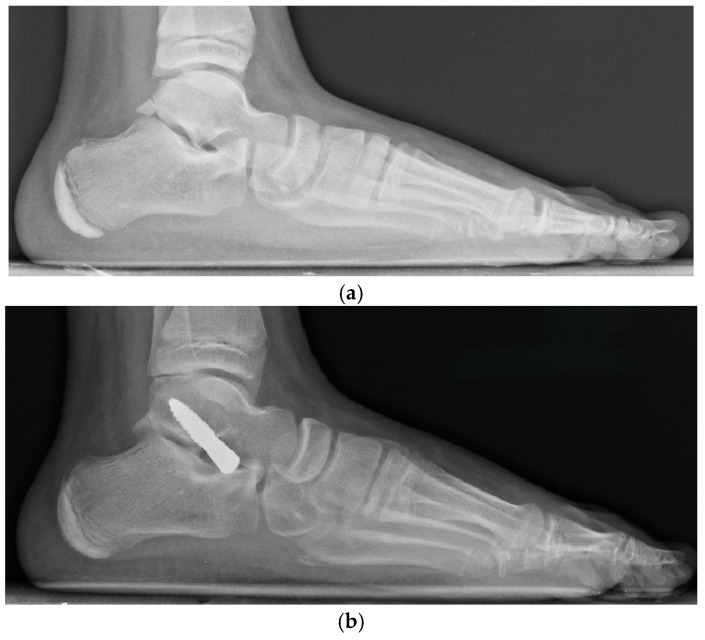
(a) Standing lateral radiograph of the left foot of an 11-year-old boy complaining of pain on the posterior aspect of the calcaneus. His diagnosis was symptomatic flatfoot. Meary’s and pitch angles measured, respectively, −4° and 14°. (b) The boy underwent surgery with an exosinotarsal talar screw, but symptoms persisted 8 months later when first seen by us. The radiolucent area present around the screw did not cause any further discomfort to the boy because tenderness was localized at the insertion of the Achilles tendon on the calcaneus. The patient was affected by Sever’s disease with a mild asymptomatic flatfoot.
